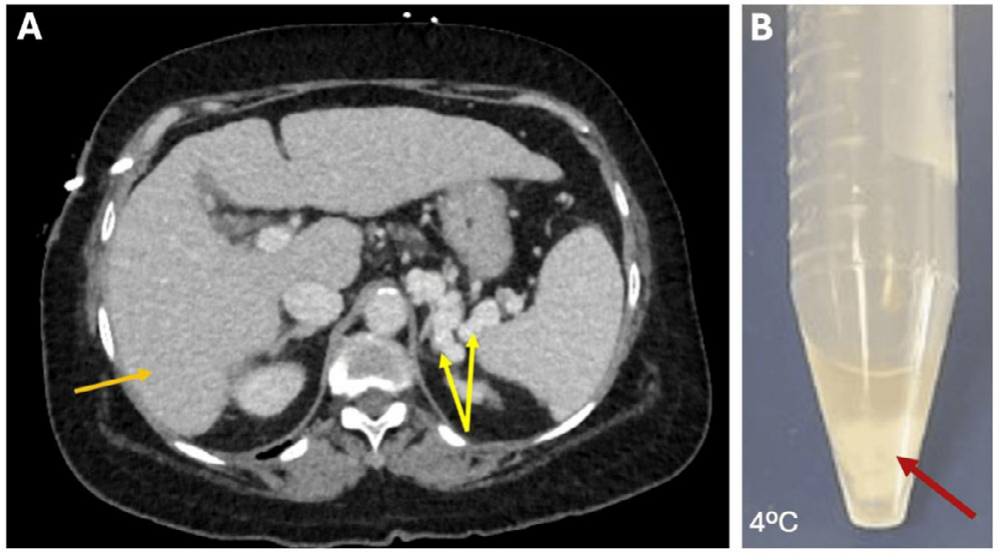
(A) Abdominal computed tomography (CT) showed the presence of the liver with cirrhotic morphology (orange arrow), as well as with multiple left upper quadrant varices consistent with portal hypertension (yellow arrows). (B) Serum sample showing a characteristic cryoglobulin pellet present at 4°C (red arrows).
